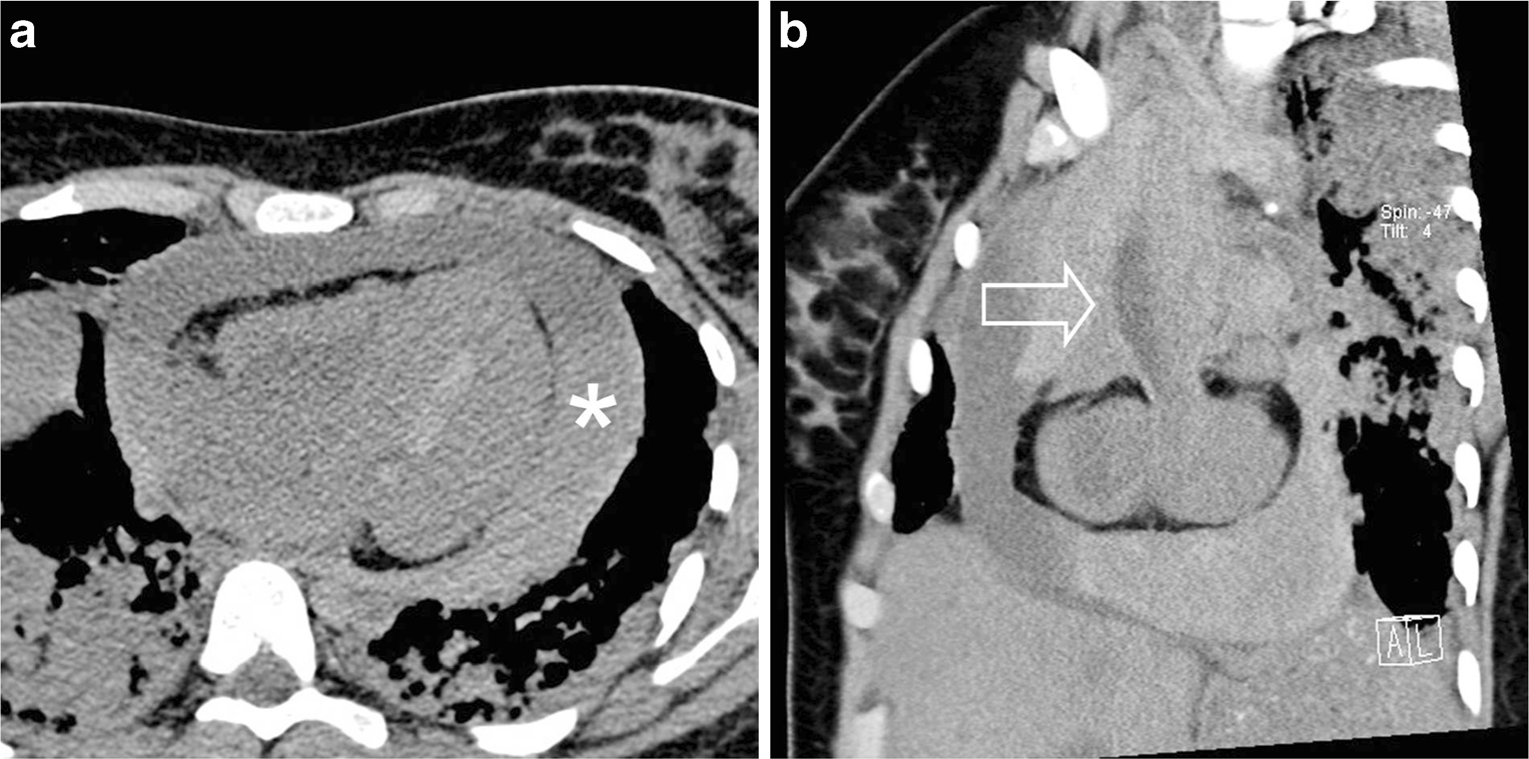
Cardiovascular cause of death. Postmortem CT in a girl age 17 years 2 months. a Axial image shows a haemopericardium (*asterisk*) with sedimentation of blood. b Sagittal oblique reconstruction shows a fusiform aortic aneurysm (*arrow*). Autopsy and genetic testing revealed a Loeys–Dietz syndrome
